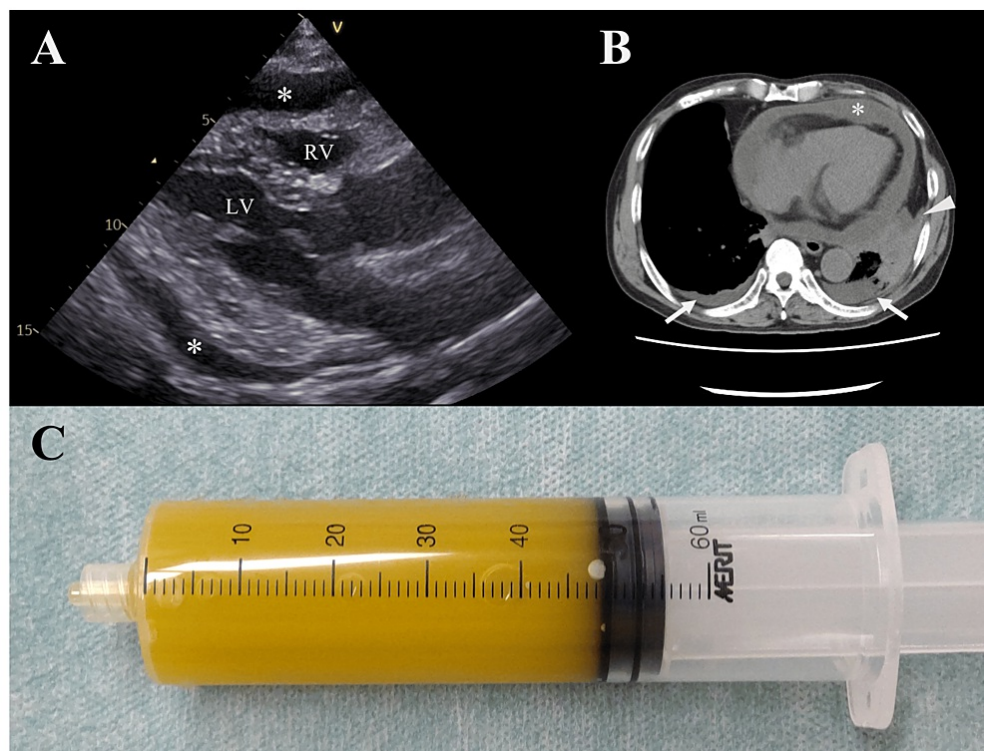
Purulent pericardial effusion. (A) Pericardial effusion on the parasternal long-axis view on echocardiography (asterisk). (B) Pleural effusion observed on chest computed tomography (arrow), atelectasis (arrowhead), and circumferential pericardial effusion (asterisk). (C) Yellowish turbid pericardial fluid collected by pericardiocentesis.
